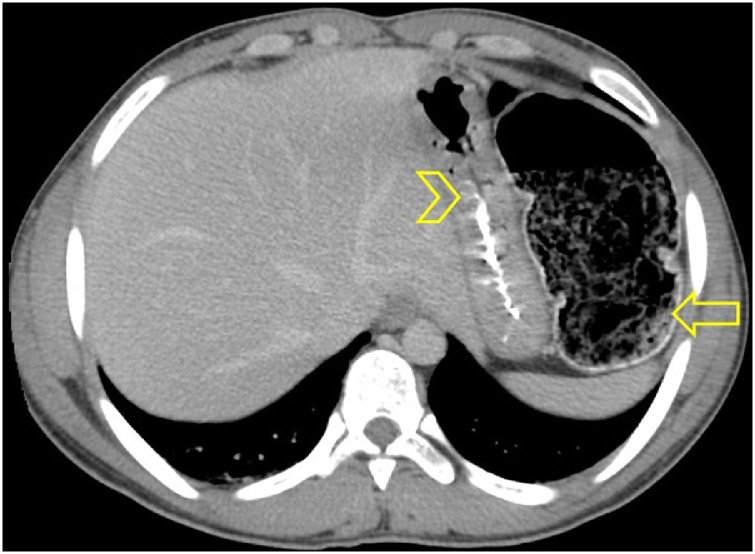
Axial CT scan of the abdomen showing distended colon (arrow) and compressed stomach (arrow head) by extrinsic compression of the colon.
